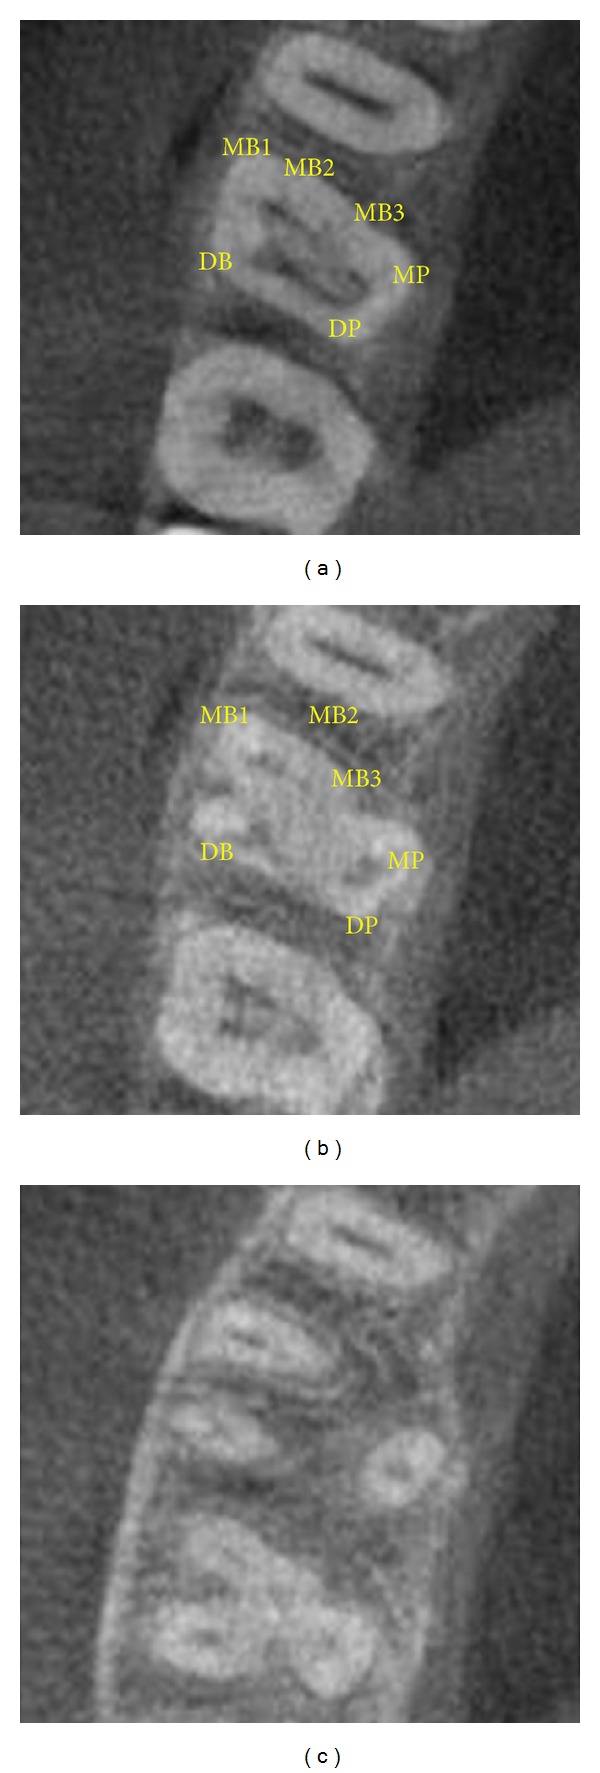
CBCT images showing distinct canal configuration.
